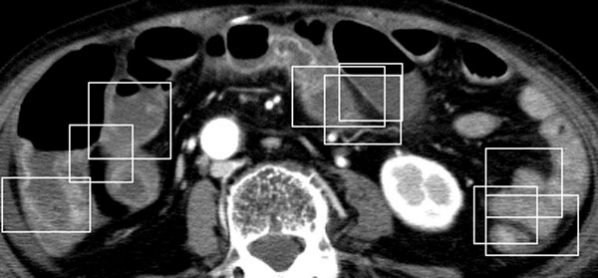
A typical result of the automated searching for the regions of interest in a CT scan of the 80 year-old female patient having ischemia: small windows indicate detected regions of interest using the proposed forward search, thus alleviating the need for the image segmentation of the intestines.
